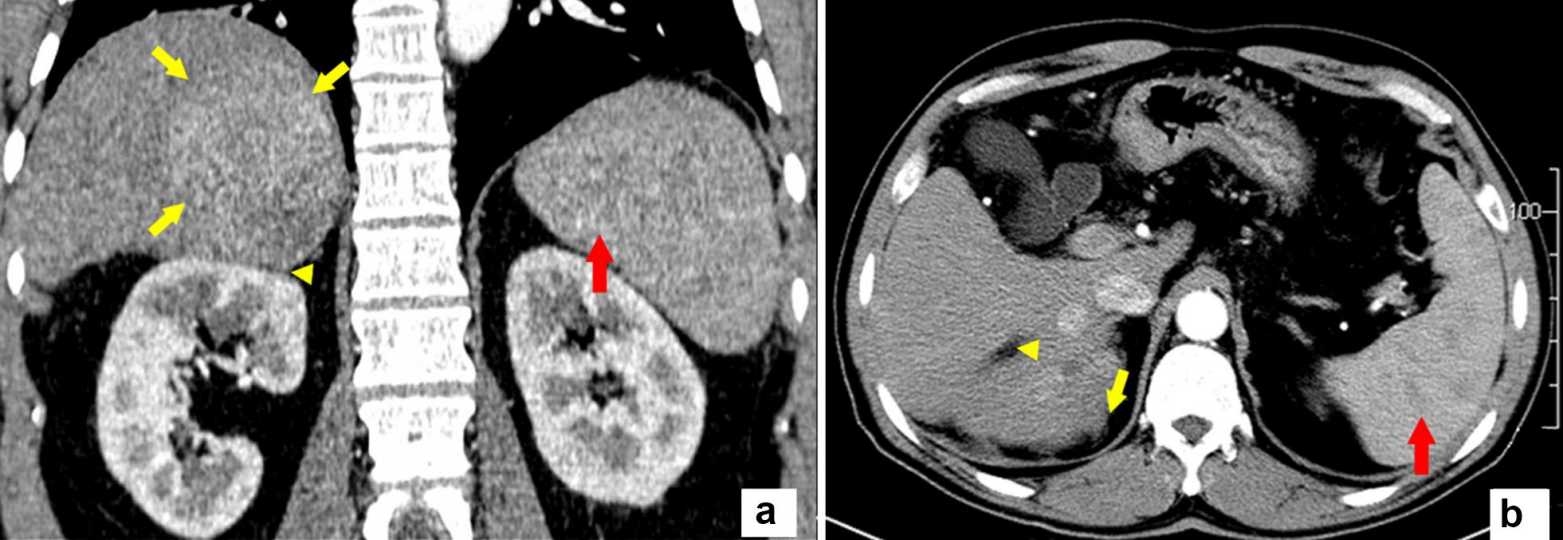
The initial computed tomography (CT) images of the patient with large hepatocellular carcinoma. The coronal a and axil b CT images in the arterial phase show a tumor (5.8 cm × 7.6 cm) occupying liver segments VI–VII (*yellow arrows*) with multifocal lesions. The inferior portion of the tumor protrudes through the liver contour adjacent to the ipsilateral renal fascia (*yellow arrowhead*). Splenomegaly can be seen as well (*red arrow*)
